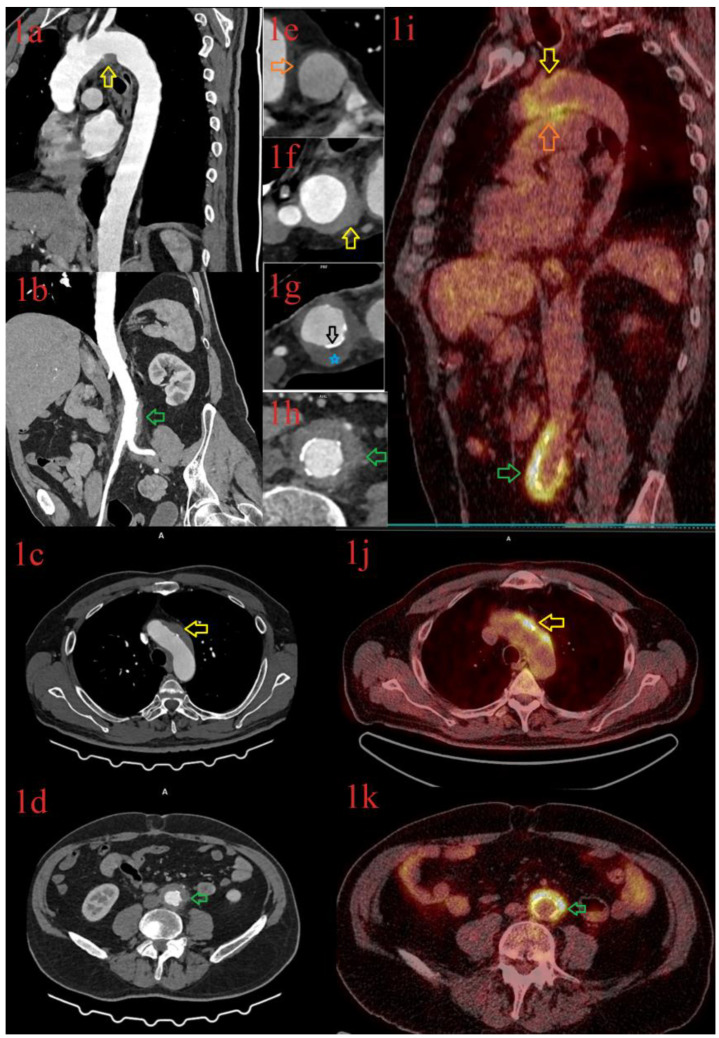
CT angiography (Images (1a–1h)) and Whole-body FDG PET-CT (Images (1i–1k)) in a 68-year-old male patient with large-vessel vasculitis showing vessel inflammation with asymmetric hypodense wall thickening (Image (1g), blue star) and intimal calcification (Image (1g), black arrow) and avid FDG uptake at the same level on the following localizations: the aortic arch (CT: Image (1a,1c,1f); PET-CT: Image (1i) I (1j); yellow arrows), pulmonary trunk (CT: Image (1e), PET-CT: Image (1i); orange arrows), and the abdominal aorta (CT: Image (1b,1d,1h); PET-CT: Image (1i) I (1k), green arrows).
